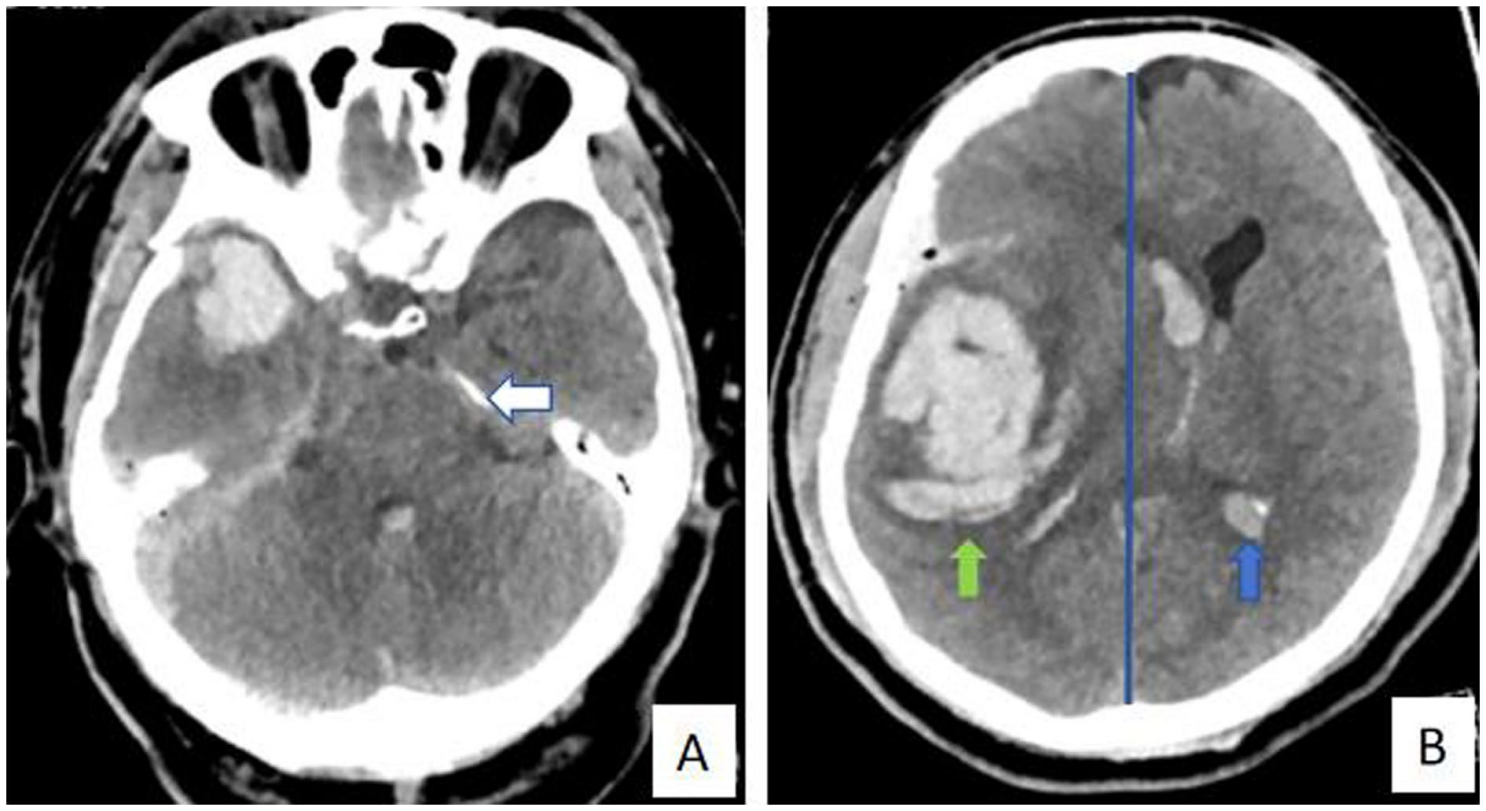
(A, B) A 60-year-old male patient with brain trauma was admitted to the hospital and underwent surgical treatment. The final prognosis was not good. Rotterdam CT score are as follows: basal cisterna status: two points for displacement (white arrow); midline displacement: one point for a shift >5 mm (blue line); ventricular hemorrhage or traumatic subarachnoid hemorrhage: one point for presence (blue arrow); and space-occupying lesions, such as hematomas or contusions: one point for presence (green arrow). The total score plus one resulted in six points, which was used to predict prognosis.
